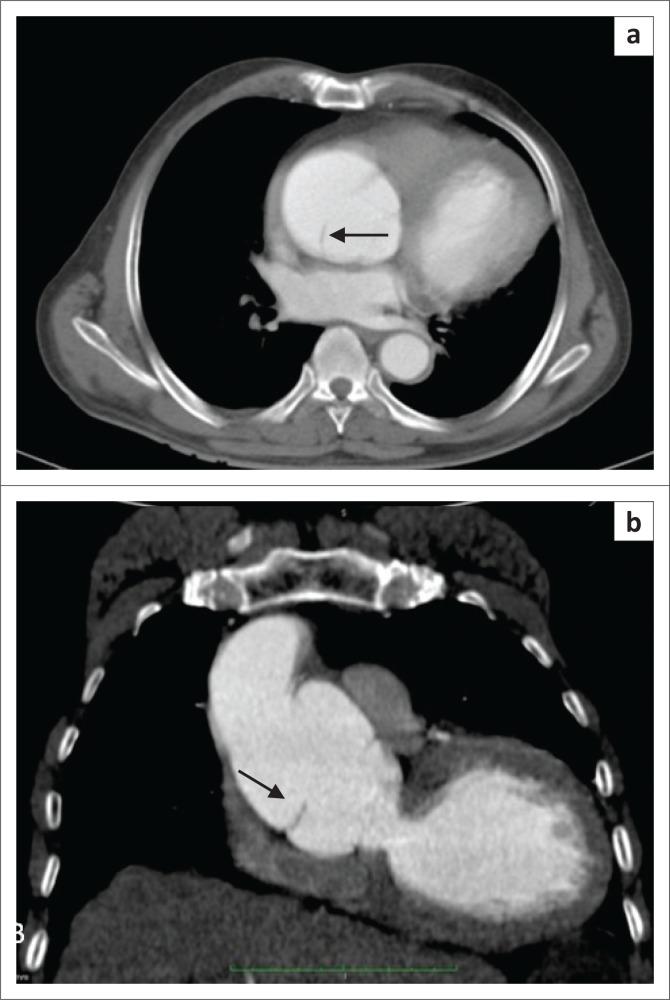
Type A chronic dissection. Contrast-enhanced axial (a) and coronal (b) computed tomography scan showing an ascending aortic aneurysm with chronic dissection. A thin linear low attenuation discontinuous dissection flap (arrow) is seen within the aneurysm.
